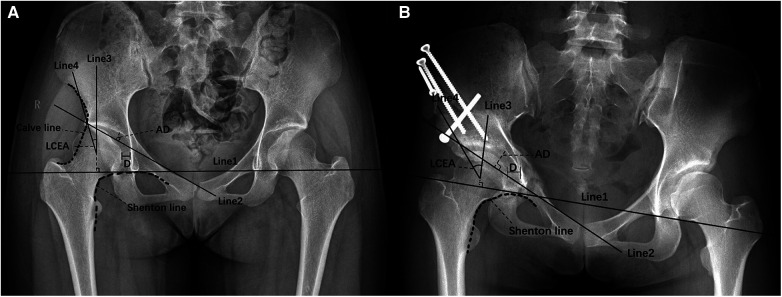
Measurement methods. (A) Preoperative pelvic anteroposterior x-ray. Line 1 is the teardrop connection. Line 2 connects the outermost point of the weight-bearing area of acetabular sclerosis and the symphysis pubis's upper corner; the AD is the greatest perpendicular distance from the acetabular roof to Line 2. Line 3 passes through the center of the femoral head and is perpendicular to Line 1, Line 4 is the connection between the center of the femoral head and the outermost point of the weight-bearing area of acetabular sclerosis, and the angle formed by Line 3 and Line 4 is the LCEA. D is the distance from the innermost surface of the femoral head to the ilioischial line. The Calve line is a continuous line between the ilium's outer edge and the femoral neck's outer edge below the anterior inferior iliac spine. The Shenton line is a continuous line between the femoral neck's inner edge and the obturator's upper edge. (B) Postoperative pelvic anteroposterior x-ray. Radiographic parameters improved significantly.
